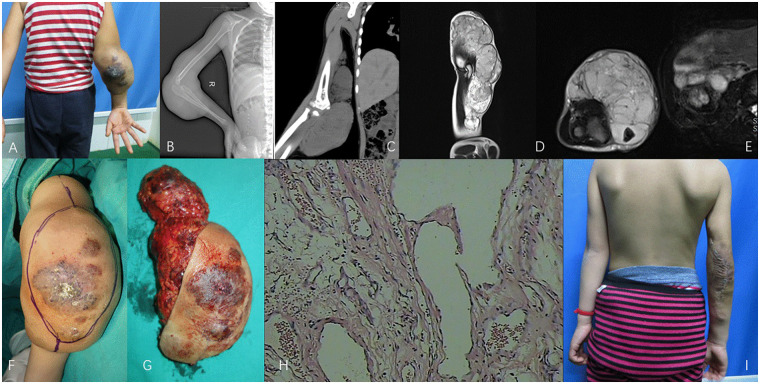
Treatment process for a 7-year-old girl with a huge lymphangioma in the right elbow. (A) Preoperative photo shows the appearance of the vascular malformation, as well as skin ulcers and scabs. (B,C) Preoperative x-rays and CT scans indicate increased density in the soft tissue of the right elbow. (D,E) Preoperative MRI showed a long T1 and T2 signal intensity in the right elbow, suggesting lymphangioma with bleeding. (F,G) Intraoperative incision design and gross view of the lesion after complete lymphangioma resection. (H) Pathological examination confirms a vascular malformation complicated with infection and bleeding, predominantly consisting of cystic lymphangioma components. (I) Postoperative appearance of the right upper limb at the 2-month follow-up.
